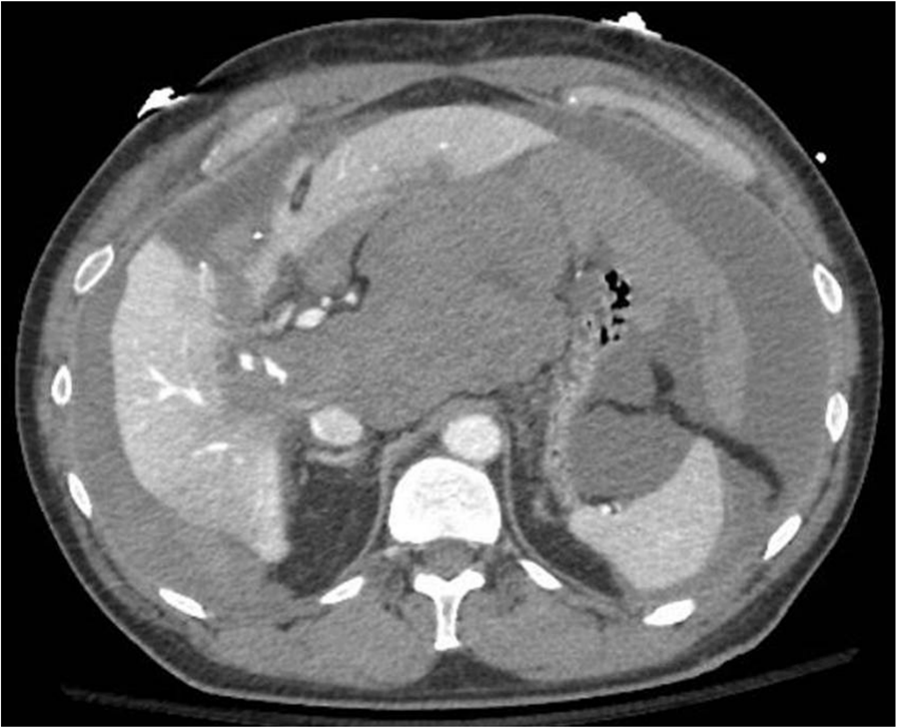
Computed tomography of the abdomen showing heterogeneous fluid (blood and ascites) in the perihepatic and perisplenic regions, and a hematoma in the lesser sac
